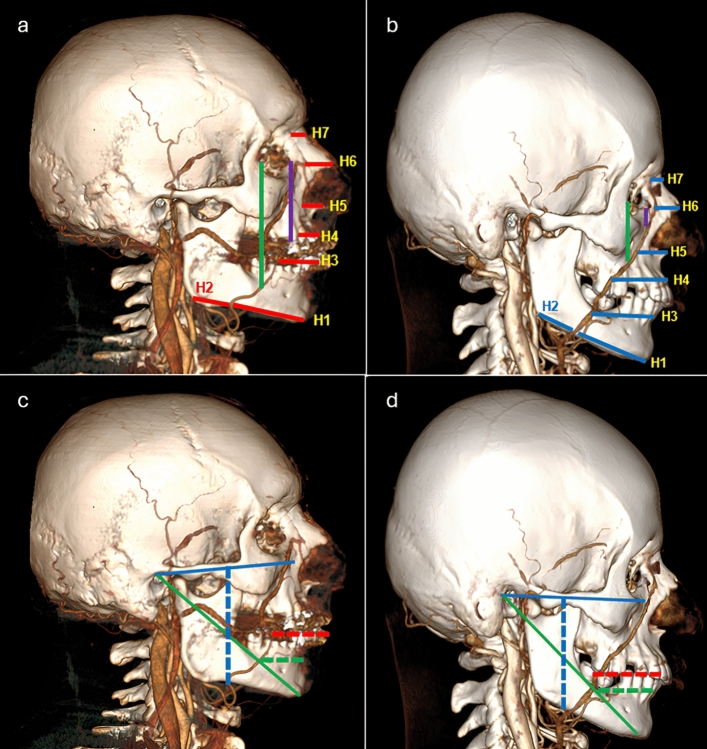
Distances of the FA and FV to anatomical landmarks and planes on three-dimensional volume rendering computed tomography angiography images. Distances of the FA (a) and FV (b) to the mental prominence (H1_MP_), mandibular angle (H2_MA_), alveolar process of the mandible (H3_APMDB_), alveolar process of the maxilla (H4_APMX_), anterior nasal spine (H5_ANS_), zygomatic arch (H6_ZA_), rhinion (H7_R_), nasion (H8_N_), medial orbital rim (purple line), and lateral orbital rim (green line). Distance between the FA (c) and FV (d) and the Frankfurt horizontal plane (blue dashed line), occlusal plane (red dashed line), and mandibular plane (green dashed line)
